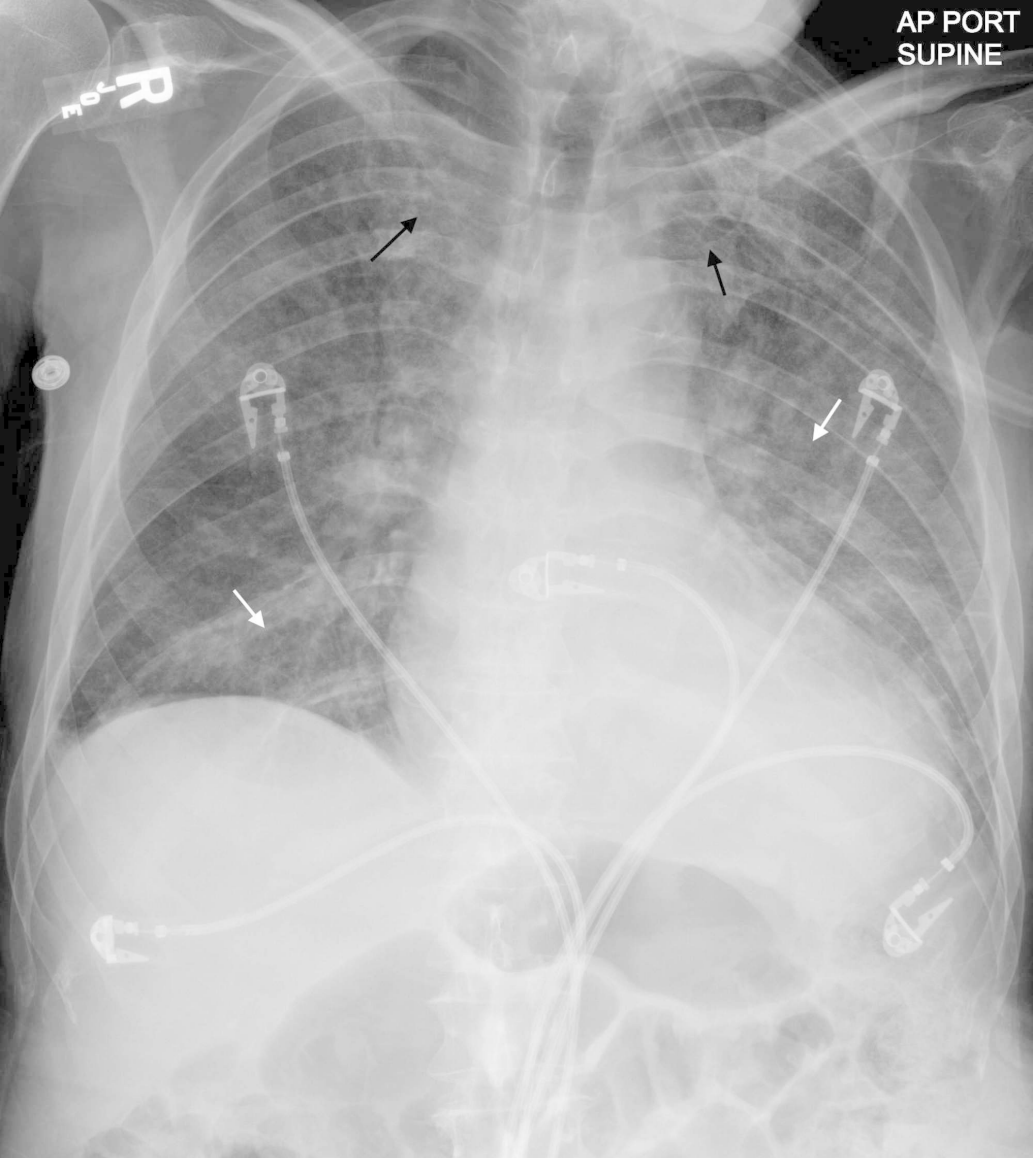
Portable anteroposterior chest radiography. Cephalization (black arrows), alveolar and reticular opacities (white arrows).
